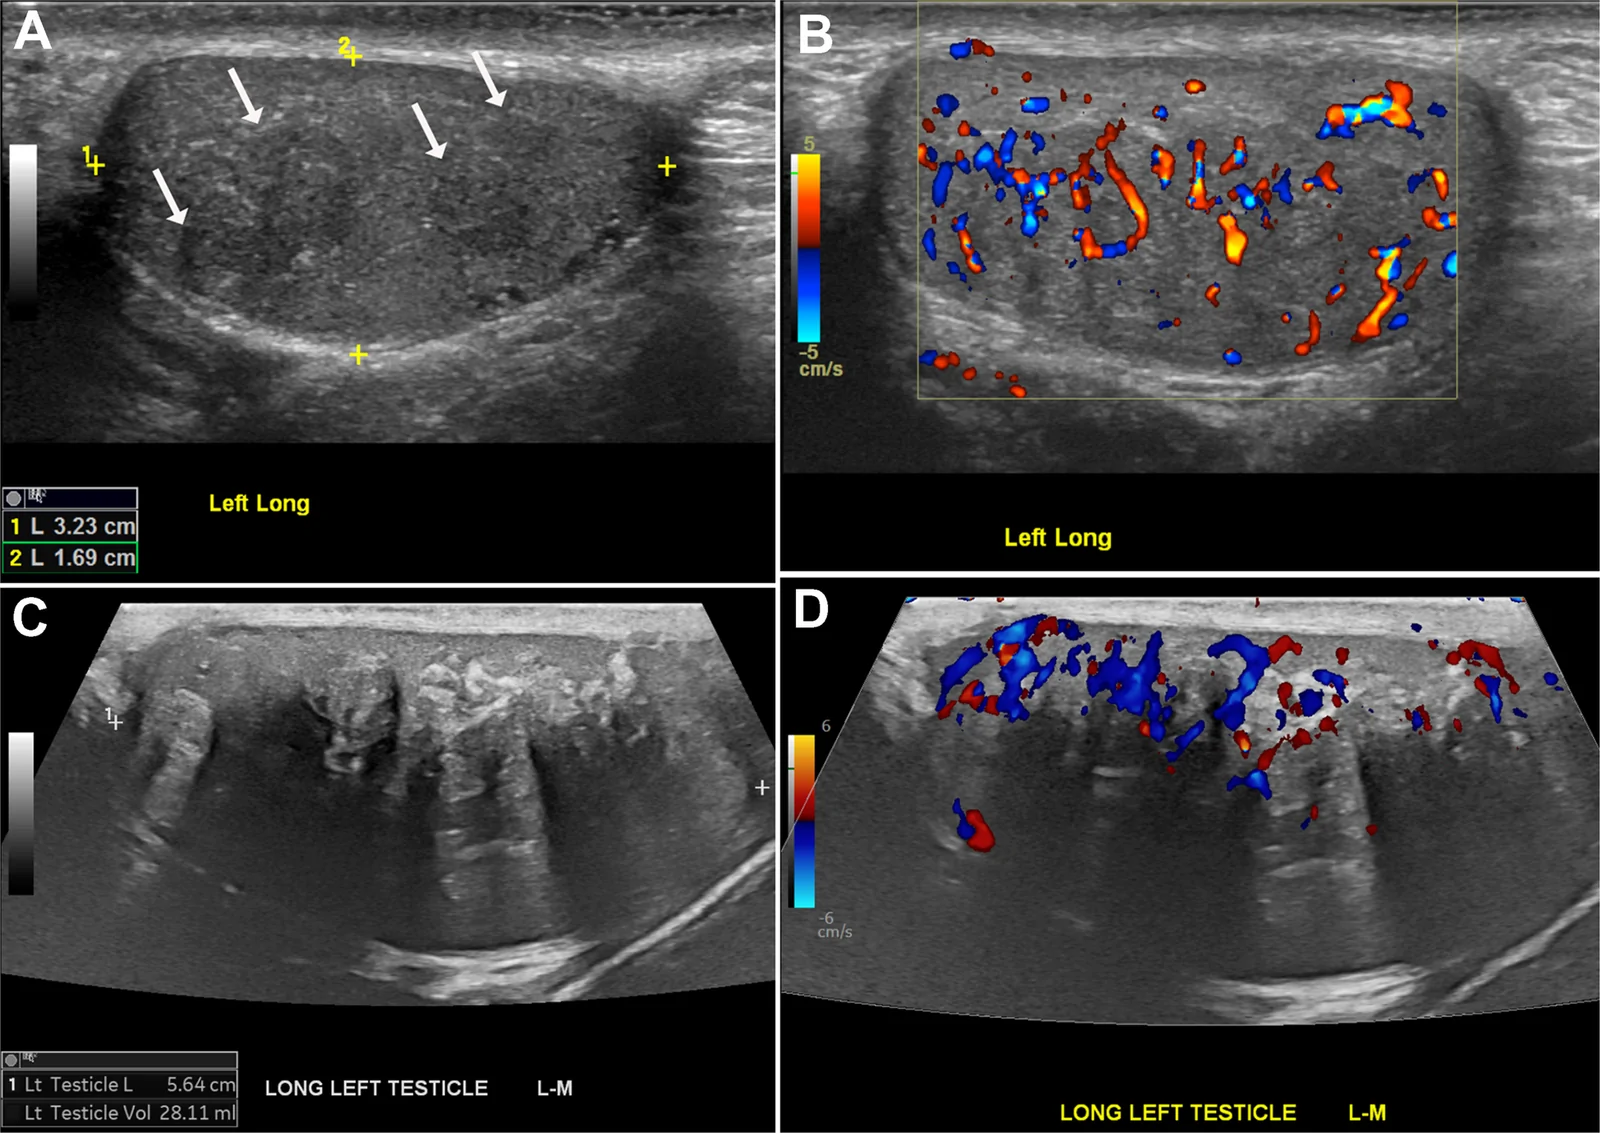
Testicular adrenal rest tumors in a 9-year-old male with history of congenital adrenal hyperplasia due to 3-beta hydroxysteroid dehydrogenase deficiency and testicular enlargement. Scrotal ultrasound shows (a) rounded scattered areas of hypoechoic tissue on grayscale image (*arrows*) and (b) hyperemia on color Doppler. c, d Repeat ultrasound performed later at age 15 shows (c) confluent areas of heterogeneity and echogenicity with posterior acoustic shadowing and (d) hyperemia on color Doppler, typical of testicular adrenal rest tumors
